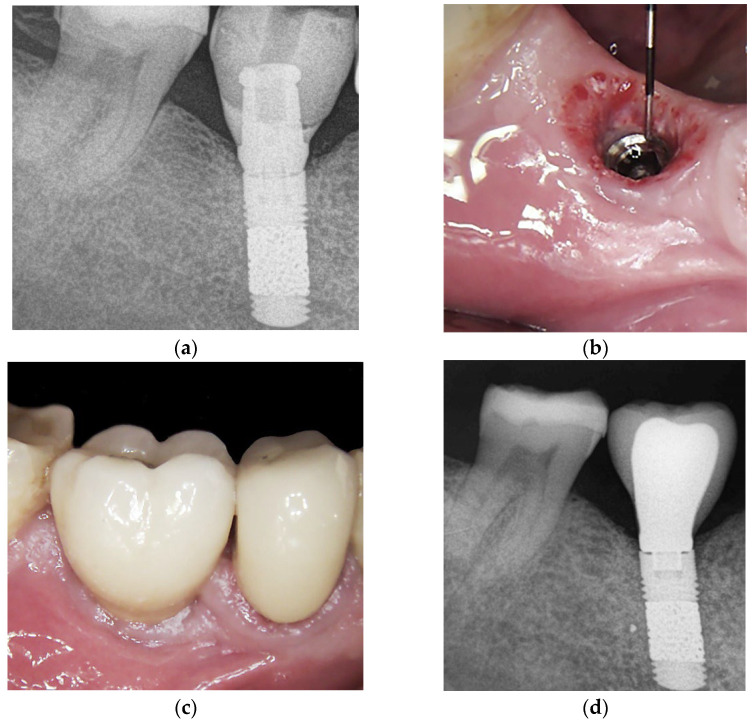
(a) Periapical X-ray after 5 months at provisional crown delivery. After 6 months, the provisional crown was removed and the soft tissue was evaluated and the ISQ values were assessed at 74 and 72 in the vestibulo-lingual and mesio-distal directions, respectively (b). The final ceramic crown was delivered (c); no bone loss was visible (d).
